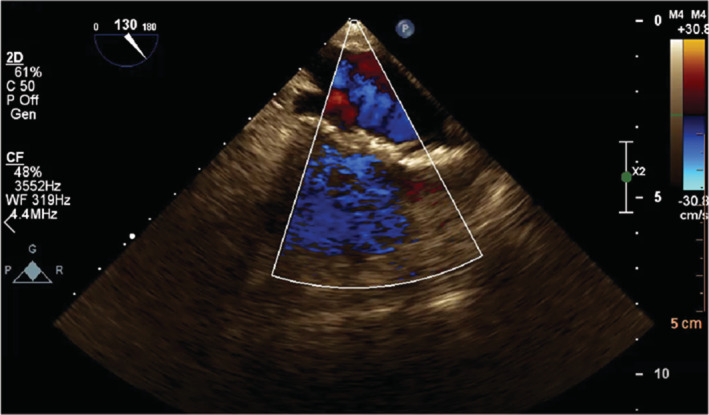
The transesophageal echocardiography in the midesophageal 130° (color mode) of the right atrium shows an elongated irregular hypermobile echodense mass.
